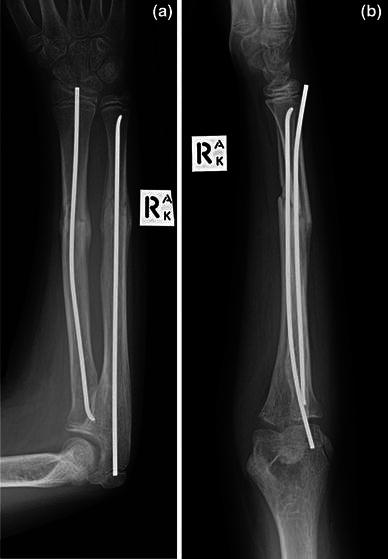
Anteroposterior and lateral radiographs representing the typical nail entry sites for all cases
